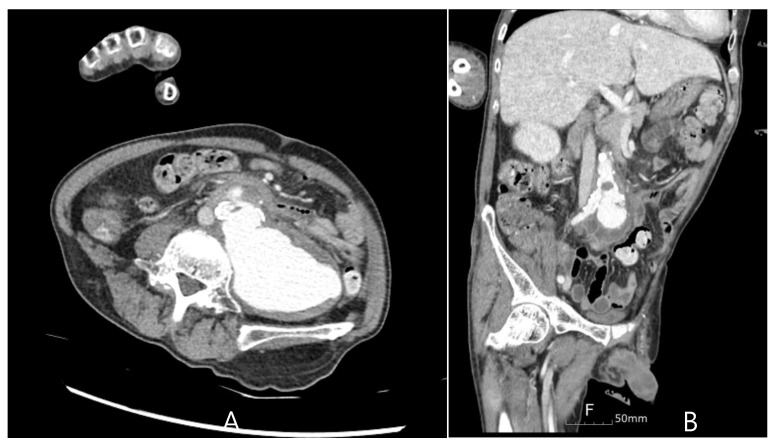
A ruptured abdominal aortic aneurysm was identified on a CT scan upon secondary admission. (A) Axial Plane, (B) Coronal Plane.
